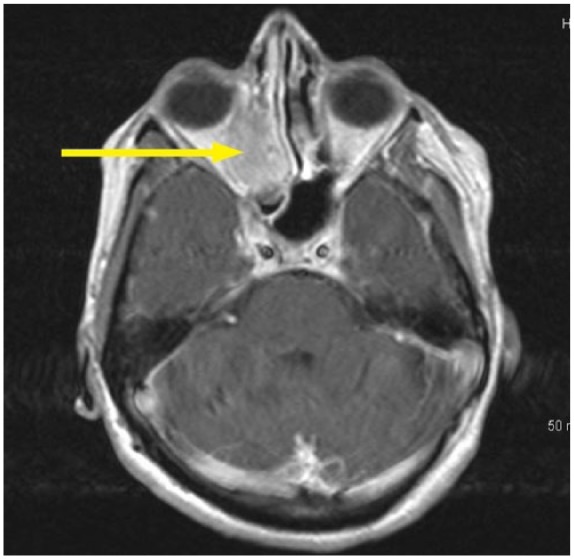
Magnetic resonance imaging right retro-orbital area with the suspected fungal mass.
